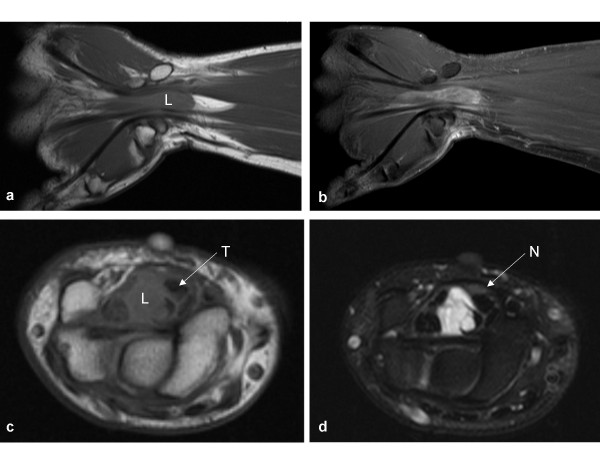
MRI of the lesion (L) shows it a) attached to the index finger profundus tendon in the coronal plane on T1 weighted imaging, b) demonstrating septal enhancement post contrast administration, c) displacing the superficialis tendons (T) to the middle and index fingers radially on axial T1 imaging, and d) showing heterogeneous T2 hyperintensity again in the axial plane, with the compressed median nerve (N) more clearly visualised.
